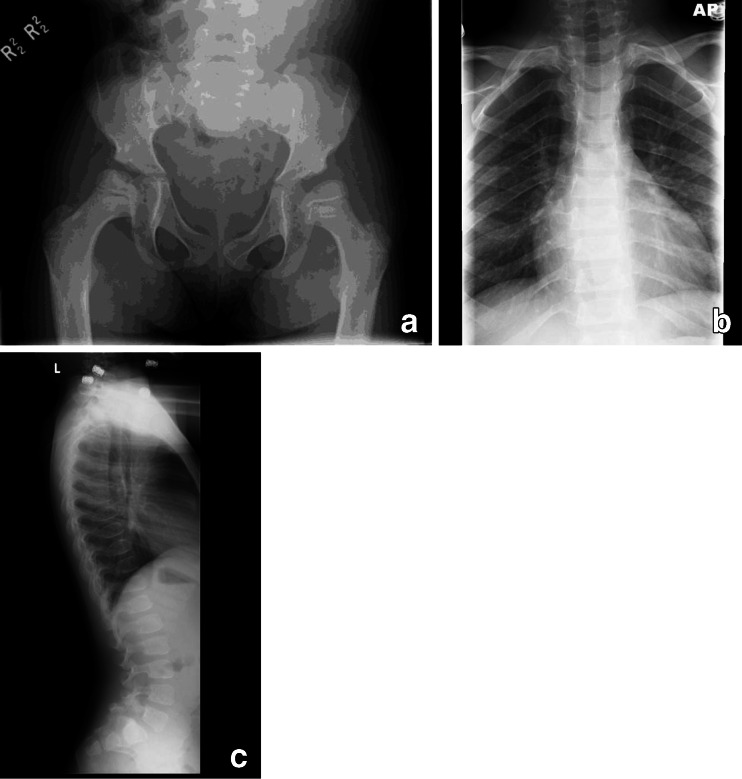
Radiographs of patient 6, female, Maroteaux-Lamy syndrome (MPS VI). a AP pelvis and hips, age 8 years: relatively normal pelvis; CFE are a little dysplastic with asymmetrical involvement; superior flattening, but erosive notching on the right. b AP spine and ribs, age 8 years: mild scoliosis (nonspecific finding); spine otherwise normal; ribs are normal without thickening/paddle shaping; clavicles are normal. c Lateral spine, age 8 years: mildly superiorly notched T9
